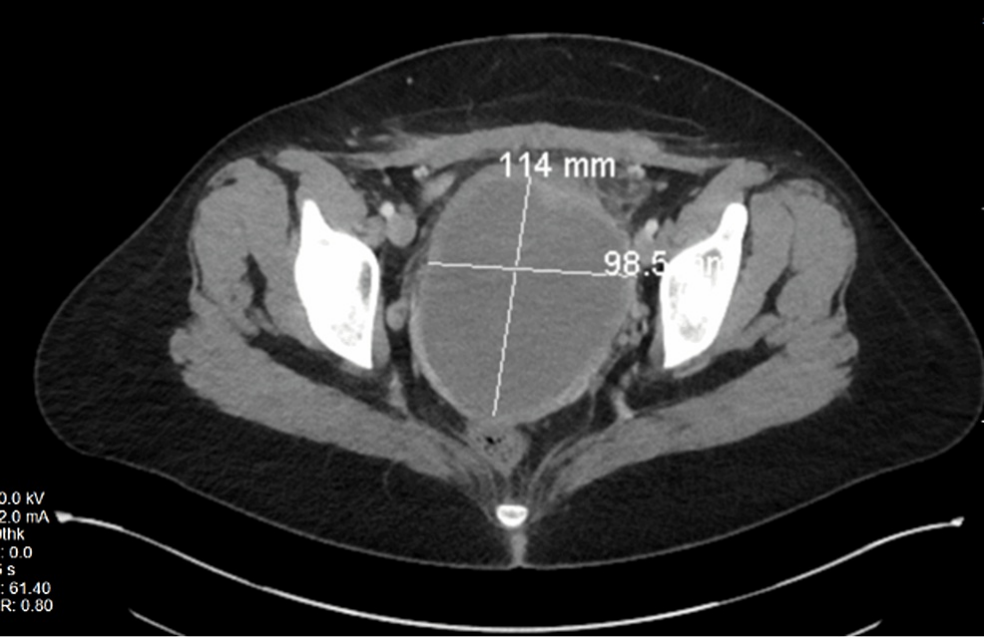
Pelvic CT showing potential mass in the pelvic cavity CT: Computed tomography Pelvic CT showing size of mass within the uterus measuring 11.4 cm x 9.85 cm. The mass takes up most of the space in the pelvic cavity, making the uterus difficult to see.
